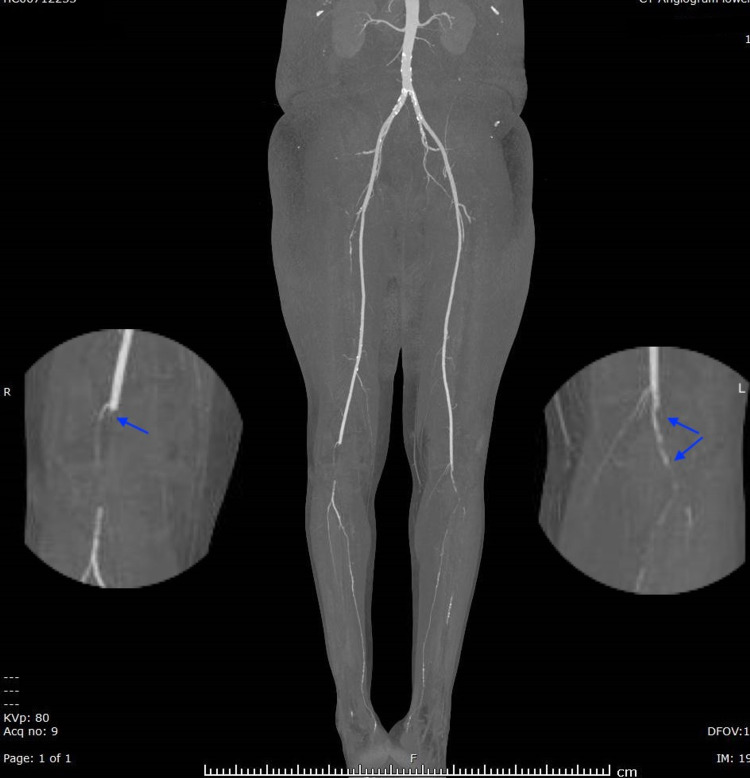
CT angiogram (anteroposterior view) showing right and left-sided popliteal artery occlusion (magnified image with blue arrows).
